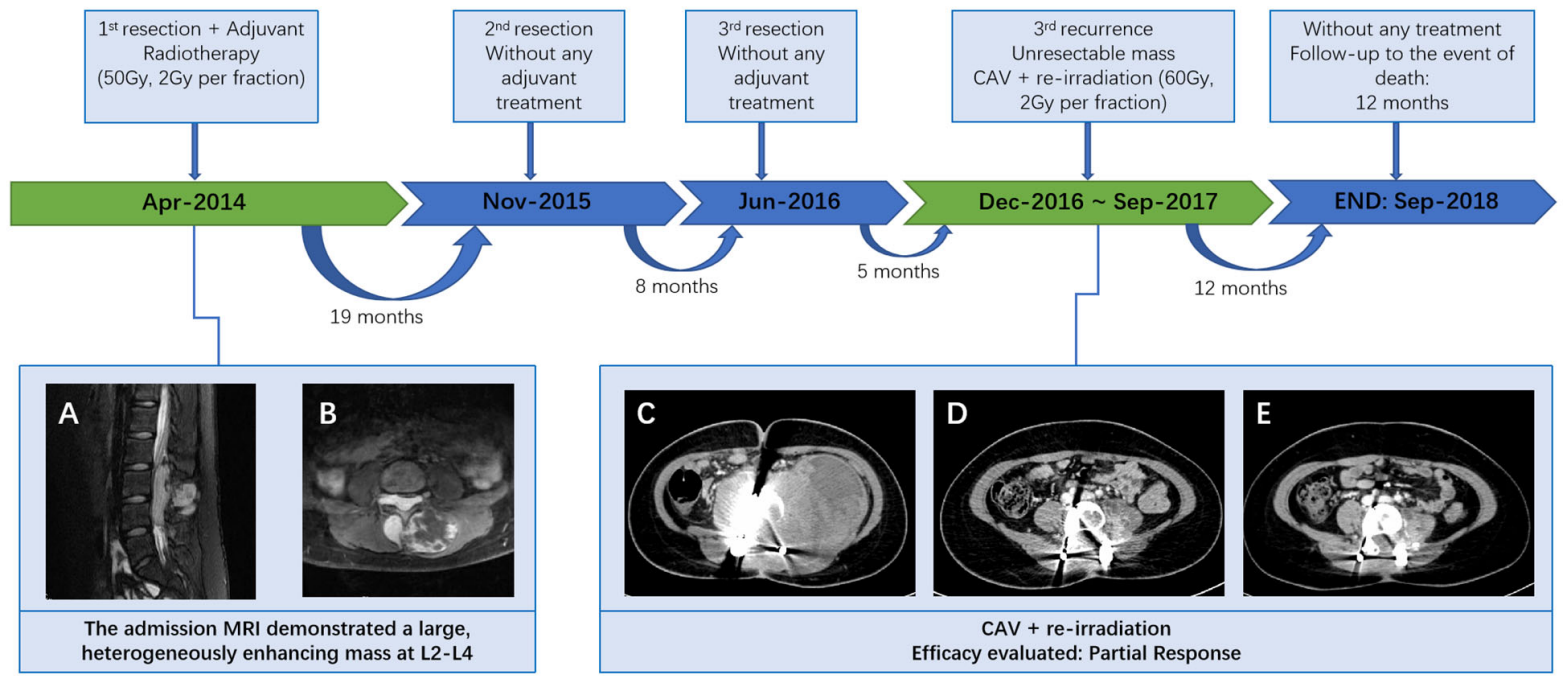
Patient treatment flow (A, B) MRI of the lumbar spine demonstrated a lesion at the L2-4 level, which invaded both the intraspinal extramedullary dura and the extraspinal canal tissue. (C) At the third recurrence, the CT scan demonstrated a giant, unevenly dense mass shadow at L3-L5 and left retroperitoneum; (D) following radiotherapy and 4 cycles of CAV, demonstrating a deep shrinkage of the mass; (E) following 8 cycles of CAV, indicating a further shrinkage.
